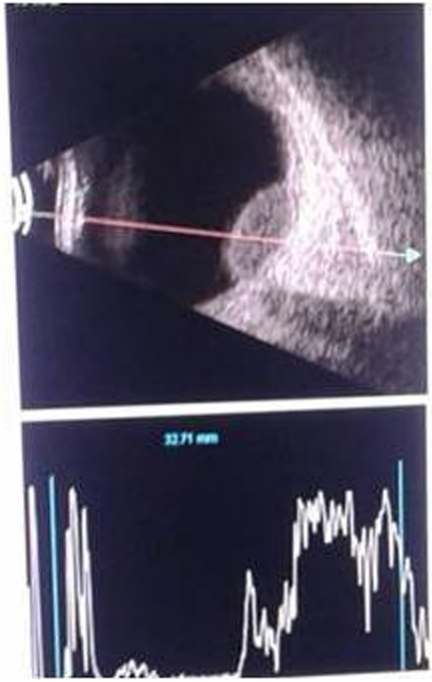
Ultrasonography shows a mass lesion of uniform echogenecity and low internal reflectivity.
